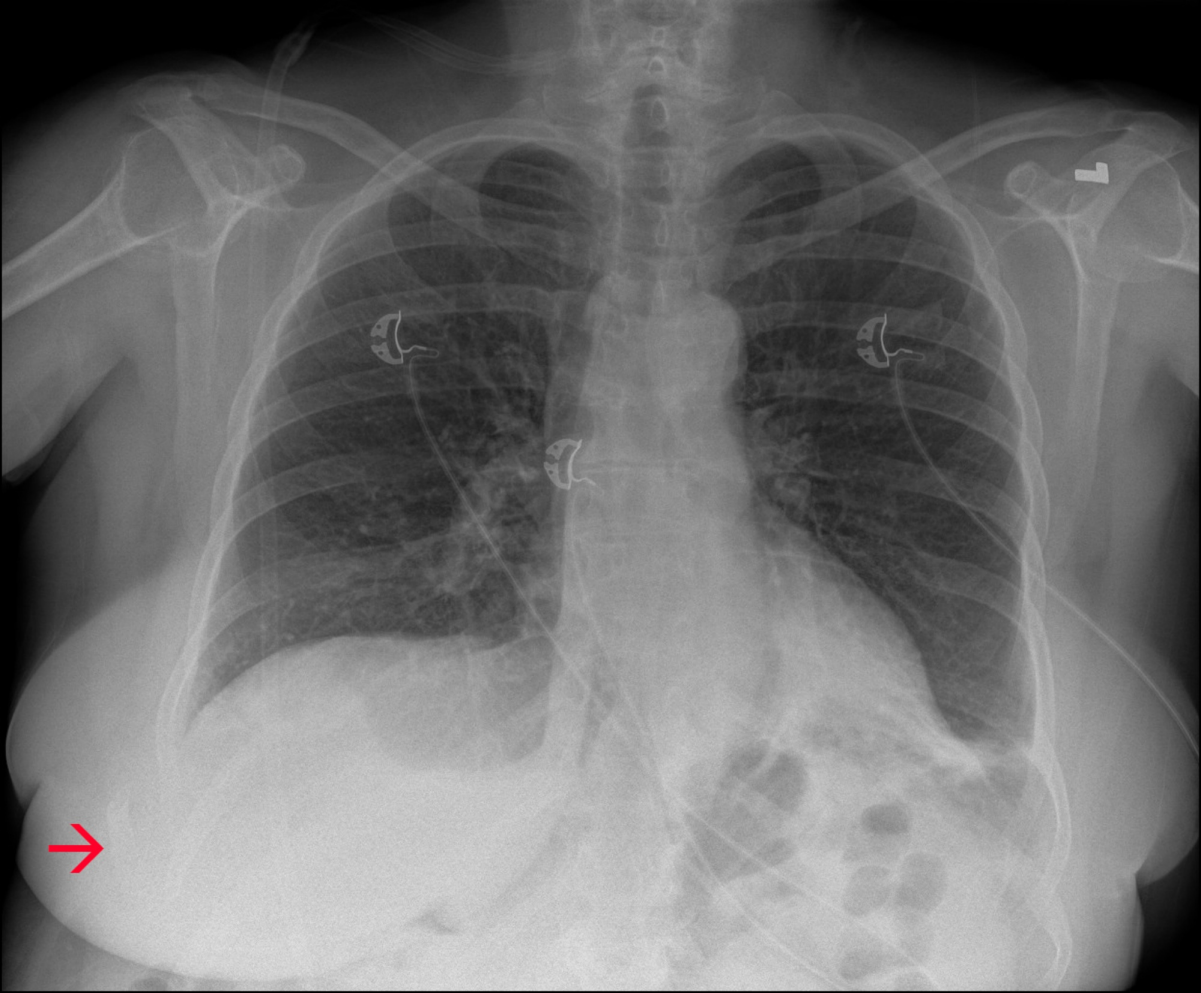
Chest X-ray. Chest X-ray revealing a right-sided ninth rib fracture (red arrow) with lateral displacement of the rib into the surrounding soft tissue. There is no evidence of pulmonary infiltrates.
